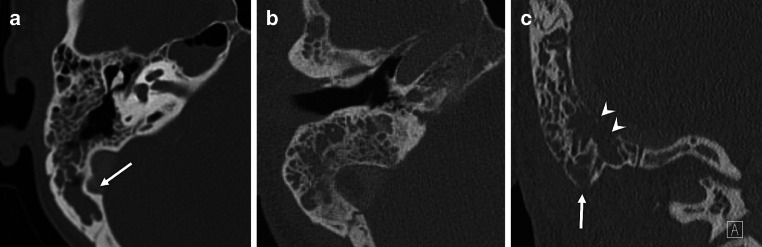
Bone destruction on CT axial (a, b) and coronal planes (c) of the right temporal bone: a no bone destruction (*arrow* marks an emissary vein), b demineralization, c definite bone destruction (*arrow* marks an external cortical defect at the tip of the mastoid and *arrowheads* mark an internal cortical defect towards the sigmoid sinus)
